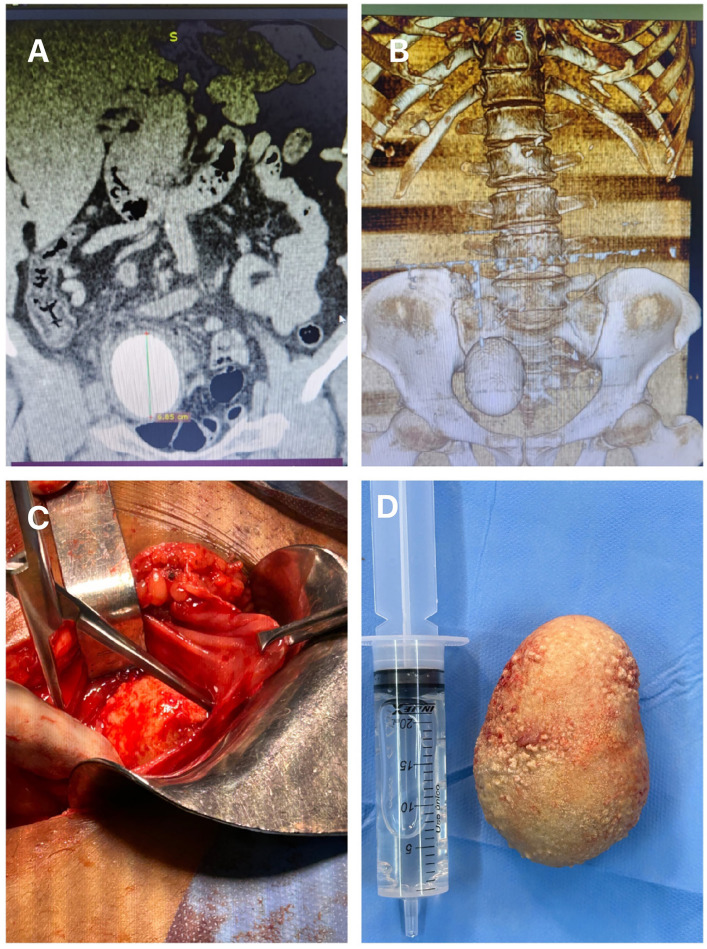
A) The figure shows the CT with the measurement of the stone inside the urinary diversion; B) In this figure we can observe a CT reconstruction showing the aspects of the Bricker stone; C) The figure shows the access to the Bricker to remove the stone and D) The figure shows the stone with 6.6cm removed after the open surgery.
